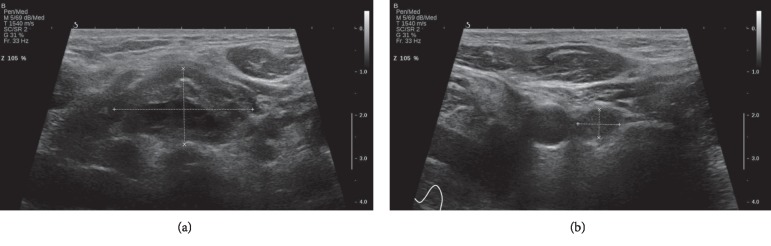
Thyroid ultrasound. Weakly hypoechoic solid nodule (2.8 × 3.2 × 1.3 cm) with a central cystic component and regular margins located in the isthmus (a). Markedly hypoechoic solid nodule (0.9 × 0.9 × 0.6 cm) adjacent to the lower pole of the left lobe (b).
